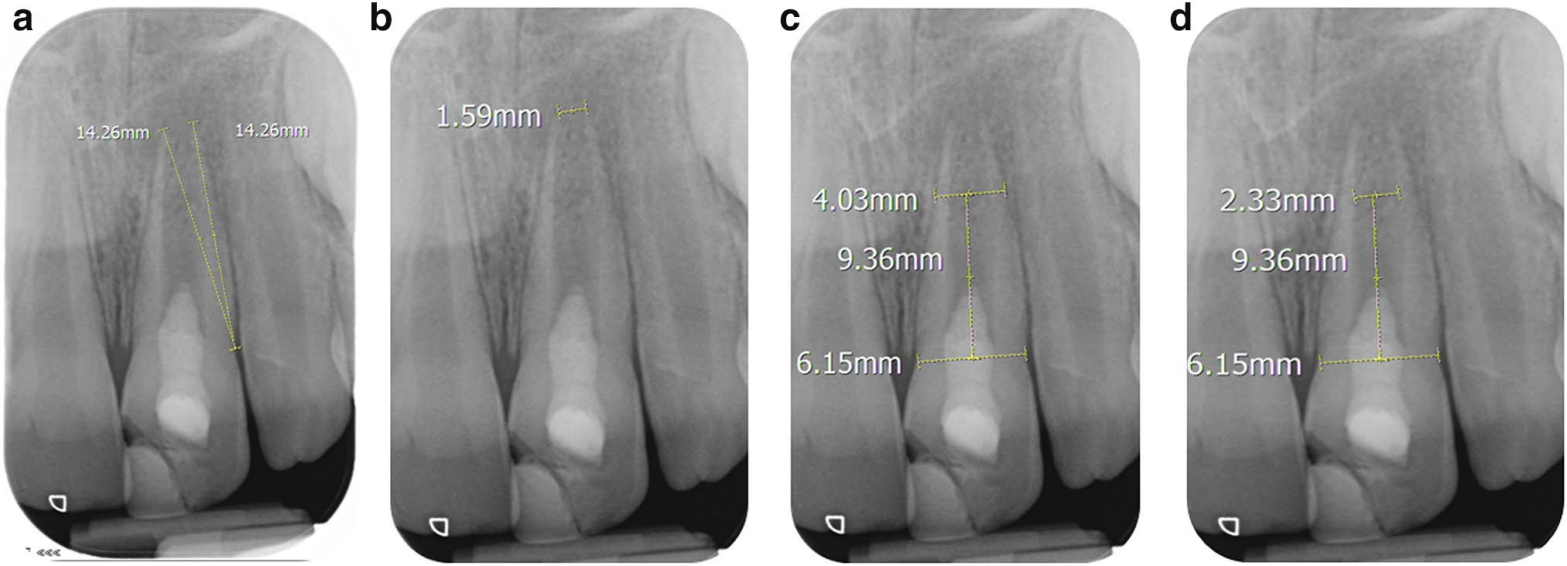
Radiographs showing root measurements (using Infinitt digital radiograph software, Seoul, Korea), a Root length: the distance between the cemento-enamel junction and the radiographic apex measured on mesial and distal sides of the root. The average of the mesial and distal root length measurements was recorded as the root length. b Apical foramen width: The distance between the mesial and distal apical ends. c, d Dentinal width: the outer root thickness and the inner pulp canal width at two-thirds root length were measured. The difference between the outer root thickness (c) and the inner pulp canal width (d) was recorded as the root dentinal wall width. Reproduced with permission from International Endodontics Journal (Nazzal et al. 2018a)
